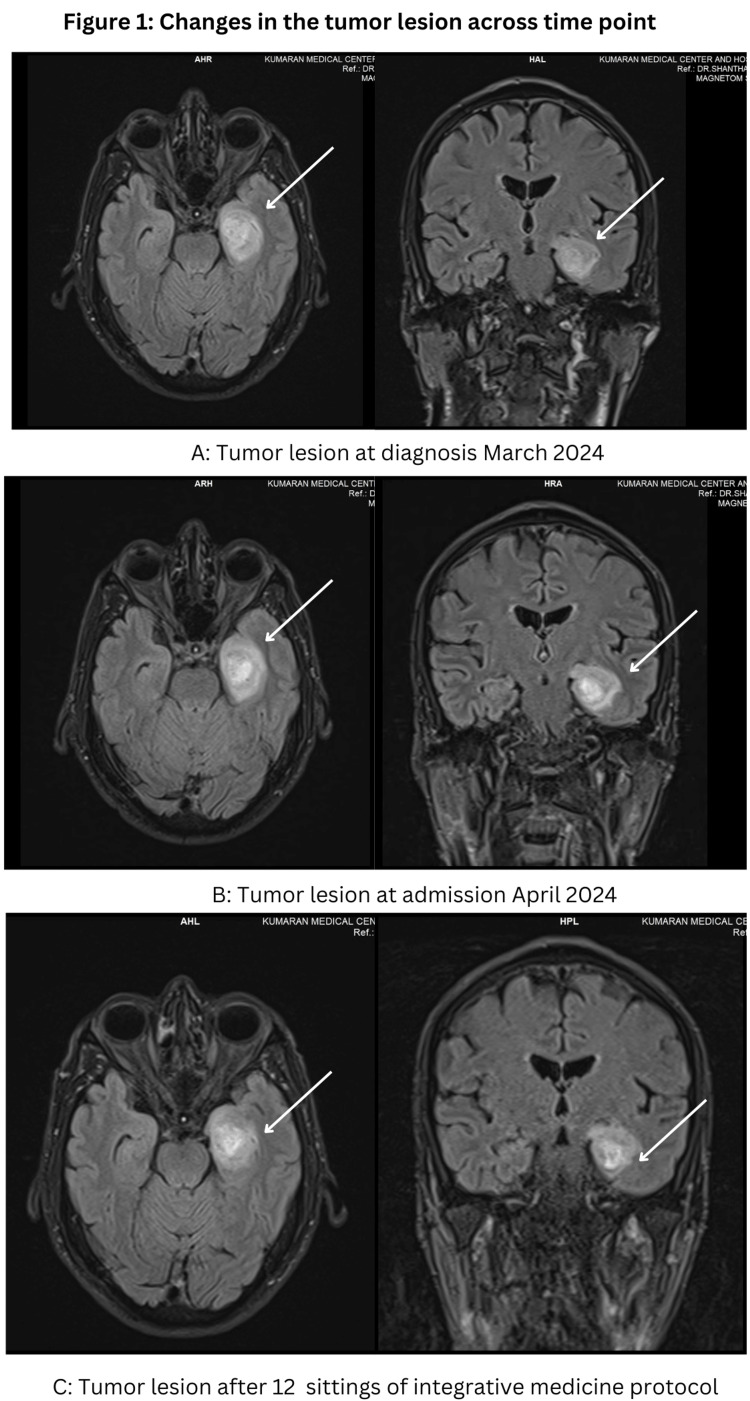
Changes in the tumor lesion over various time points. Images (A) and (B) show ill-defined mass lesions with internal necrotic areas, lobulated margins, and internal hemorrhagic areas in the left medial temporal lobe, involving uncus, amygdala, and head of the left hippocampus, measuring 3.6 x 2.9 x 2.5 cm. Image (C) shows T2/fluid-attenuated inversion recovery (FLAIR) heterointense peripherally enhancing lesion measuring 3.4 x 2.7 x 2.5 cm, seen in the left medial temporal lobe with mild surrounding edema.
